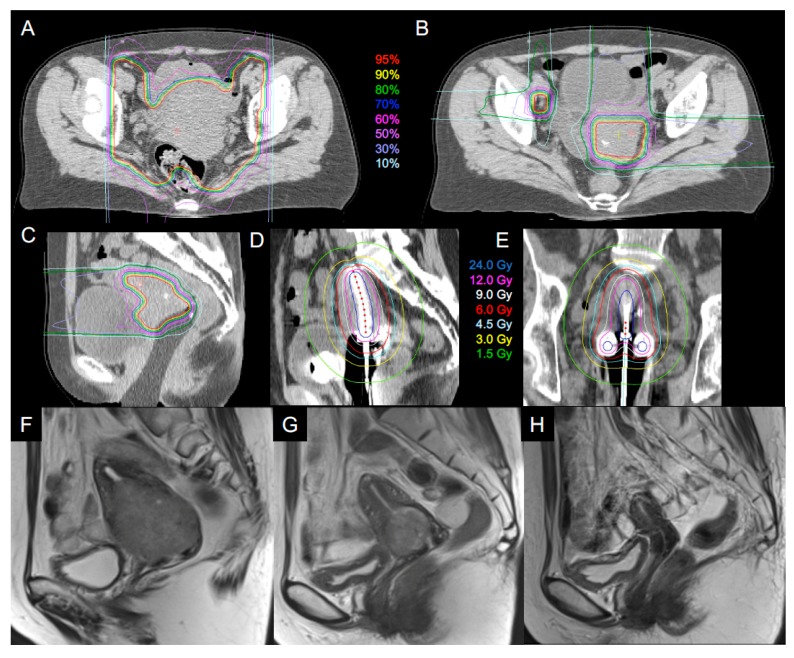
A 57-year-old patient with uterine cervical adenocarcinoma was treated with carbon ion radiotherapy (C-ion RT) and three-dimensional (3D) image-guided brachytherapy (3D-IGBT). This figure shows the dose distribution of C-ion RT for whole-pelvis. (A) Boost to primary tumor and positive lymph nodes; (B) Dose distribution of C-ion RT boost in sagittal image; (C) A vaginal immobilization device was inserted to fix the upper vaginal position and to separate the rectum from the cervical tumor; Dose distribution of 3D-IGBT in a sagittal image (D) and a coronal image (E); T2-weighted magnetic resonance imaging before treatment (tumor size, 6.4 cm) (F); at the end of treatment (G); and 36 months after treatment (H).
